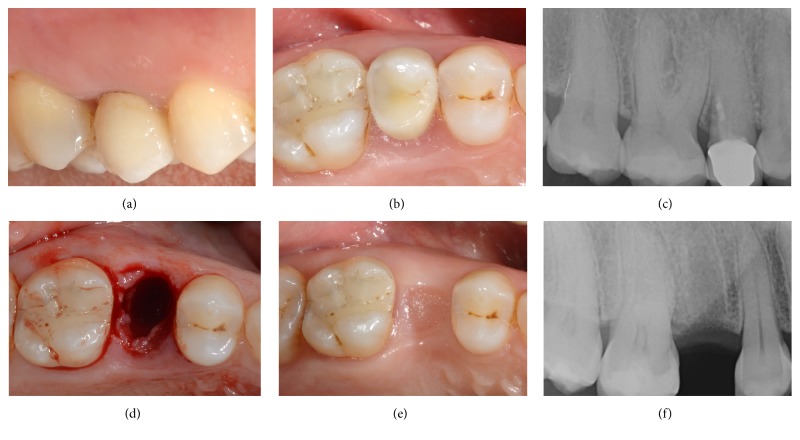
Delayed implant placement of a maxillary second premolar (#15). Preoperative clinical picture, lateral view (a); preoperative clinical picture, occlusal view (b); periapical radiograph before tooth extraction (c); alveolar ridge immediately after extraction (d); alveolar ridge after 3 months of healing (e); periapical radiograph after 3 months of healing (f).
